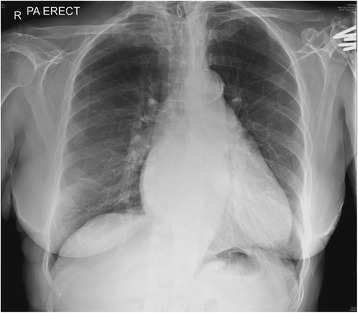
Patient’s chest X-ray on admission showing features consistent with interstitial edema
